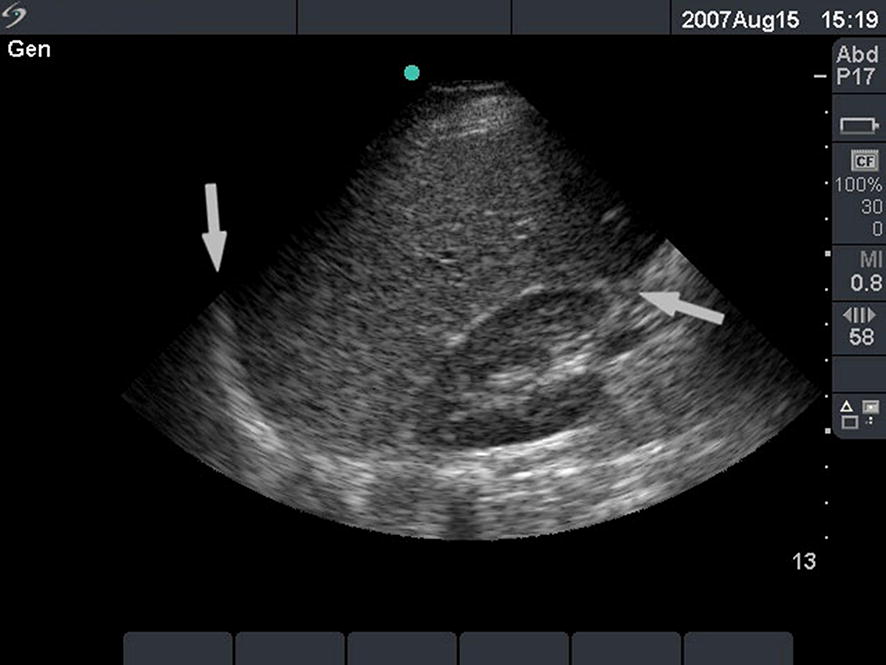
Normal hepatorenal recess. A normal ultrasound image of the hepatorenal recess (Morison’s pouch). A phased-array cardiac transducer was used with the abdominal settings. The left arrow indicates the diaphragm. The right arrow indicates the hepatorenal recess. The liver is shown above this line and the right kidney below
